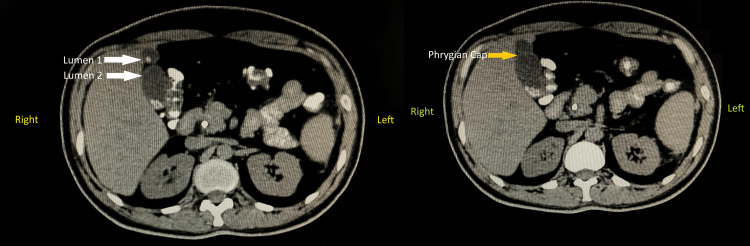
Computed tomography showing abnormal gallbladder and Phrygian cap Lumen 1 and Lumen 2 are highlighted by the white-coloured arrows, as the computed tomography image (left side) shows an abnormal gallbladder. The yellow-coloured arrow highlights the Phrygian cap in the given computed tomography image (right side).
